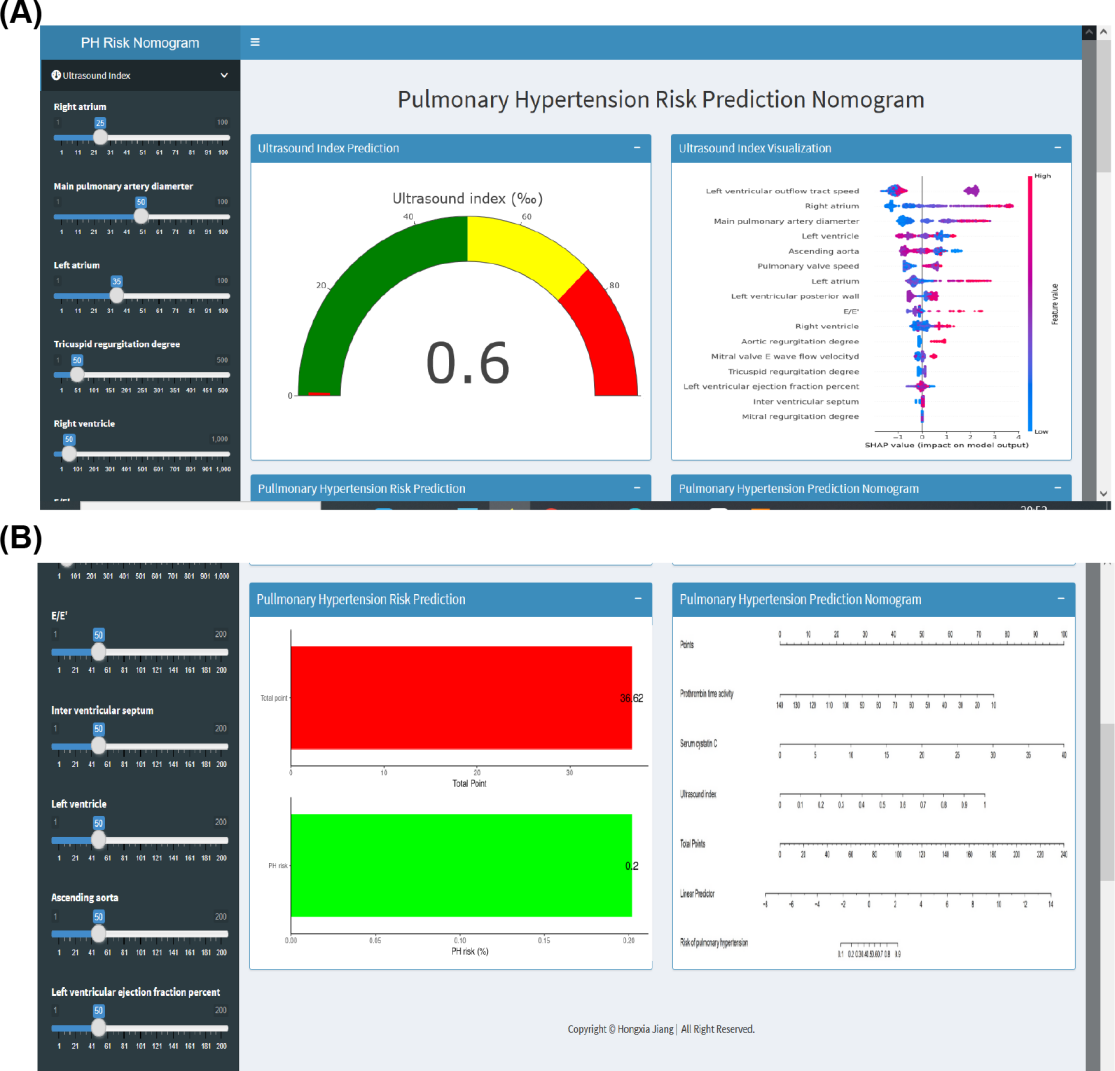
Screenshots of the nomogram web calculator. (A) Screenshot of the web calculator showing the calculated PH probability based on ultrasound index input and SHAP-based interpretation of feature importance for the ultrasound index variables. (B) Screenshot of the web calculator displaying the total points calculated via nomogram and the corresponding PH risk probability. E/E’: ratio of mitral valve early diastolic inflow velocity (E) to mitral annulus early diastolic velocity (E’); PH: pulmonary hypertension; SHAP: Shapley Additive Explanations.
